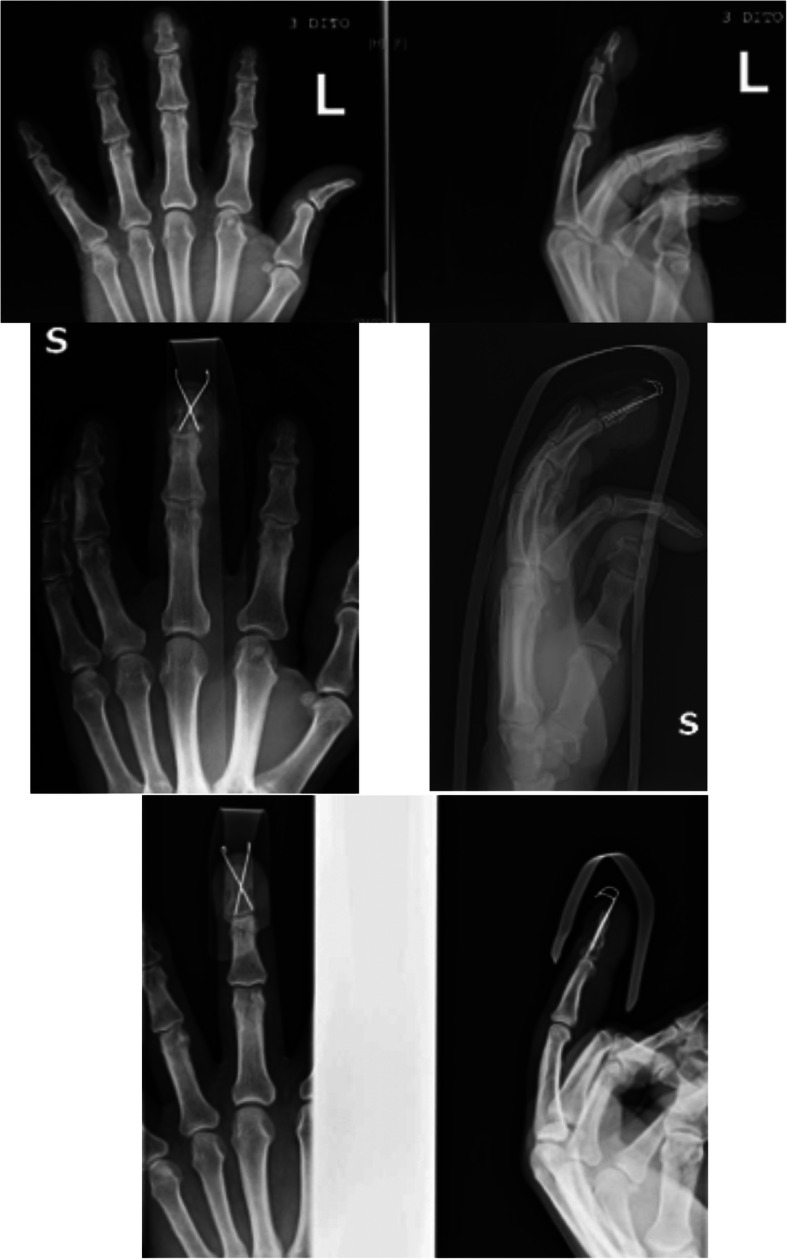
Male patient, 50 years old with crush injury and fracture dislocation of the shaft of the middle finger and dorsal soft tissue lesion. Crossed 0.8 mm k-wires fixation was performed. X-rays 50 days postoperatively depict fracture union
